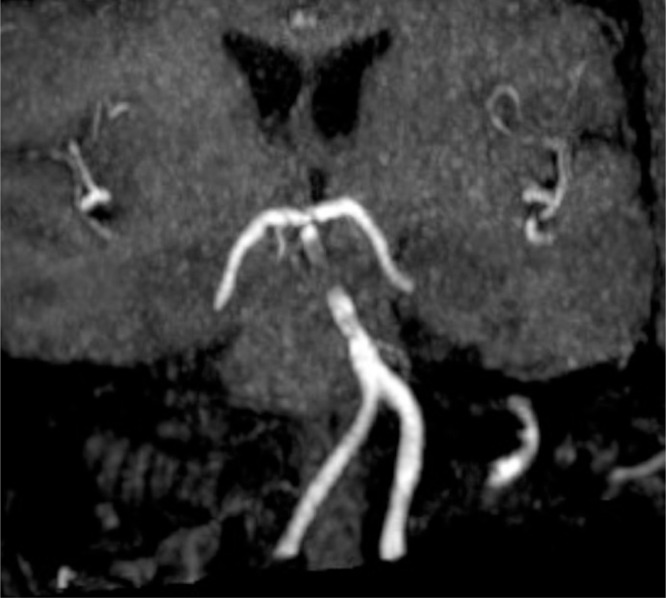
One-month follow-up MRI without obstruction within the stent.
